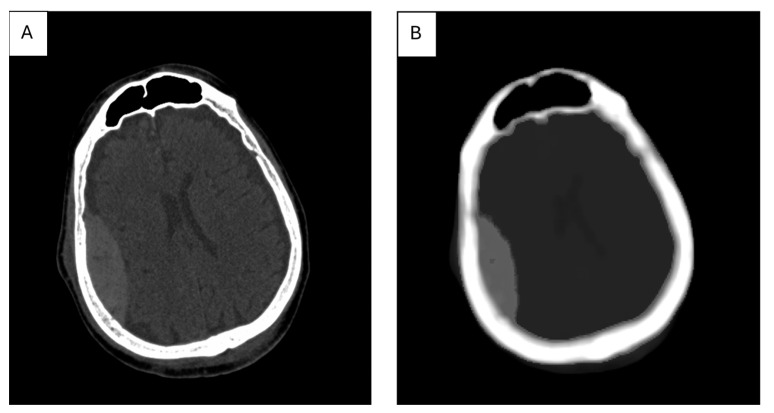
CT image of a brain cross-section with a marked stroke area (A); AngularSecondMoment feature map calculated for this image, in which a 15 × 15-pixel window was used (B).
